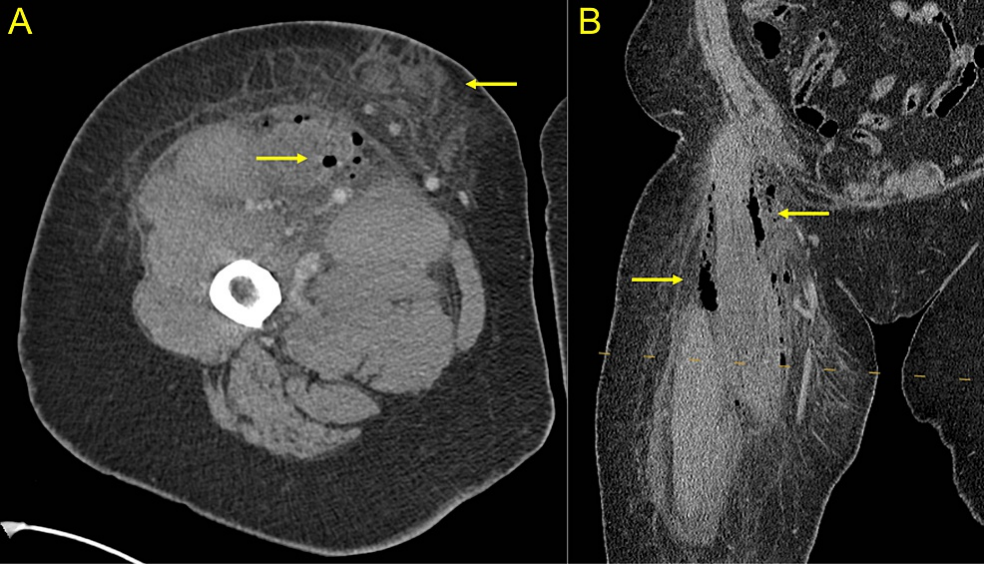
Computed tomography (CT) of the right lower extremity CT scan of the right lower extremity reveals soft tissue gas and edema (yellow arrows). (A) Axial view. (B) Coronal view.
